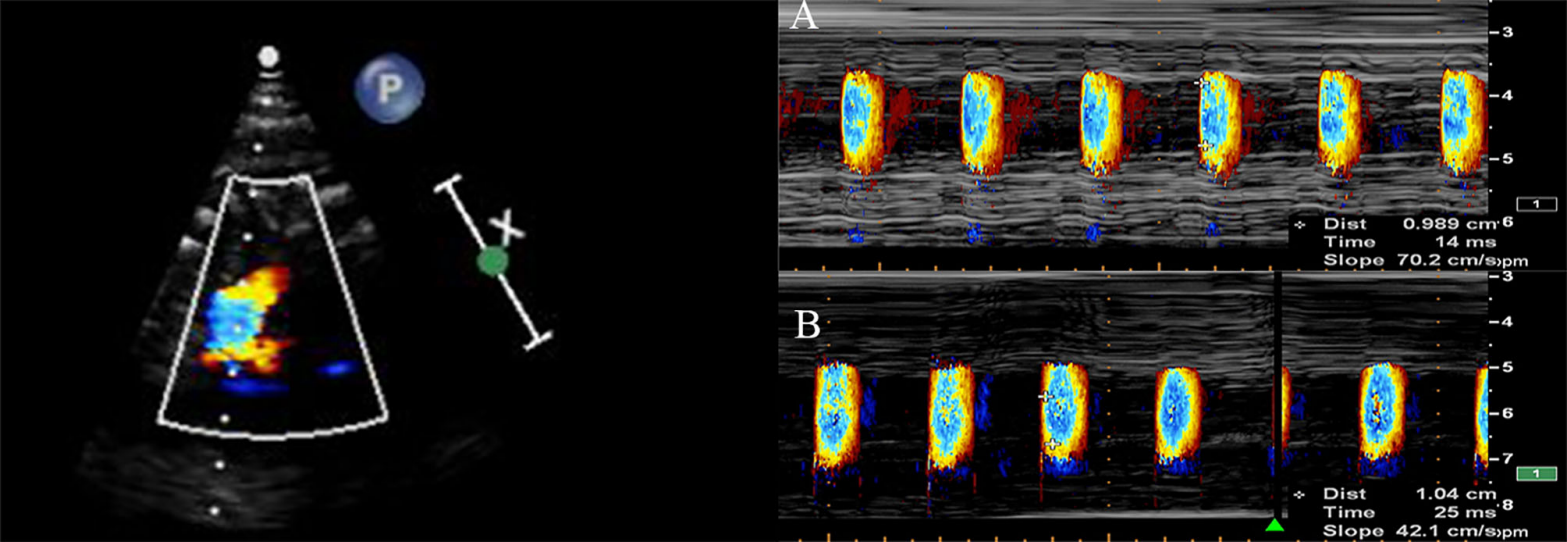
The aortic propagation velocity evaluated by color M-mode echocardiography display across the aorta arch from the sagittal view in a normal fetus (A) and in a fetus exposed to GDM (B).
